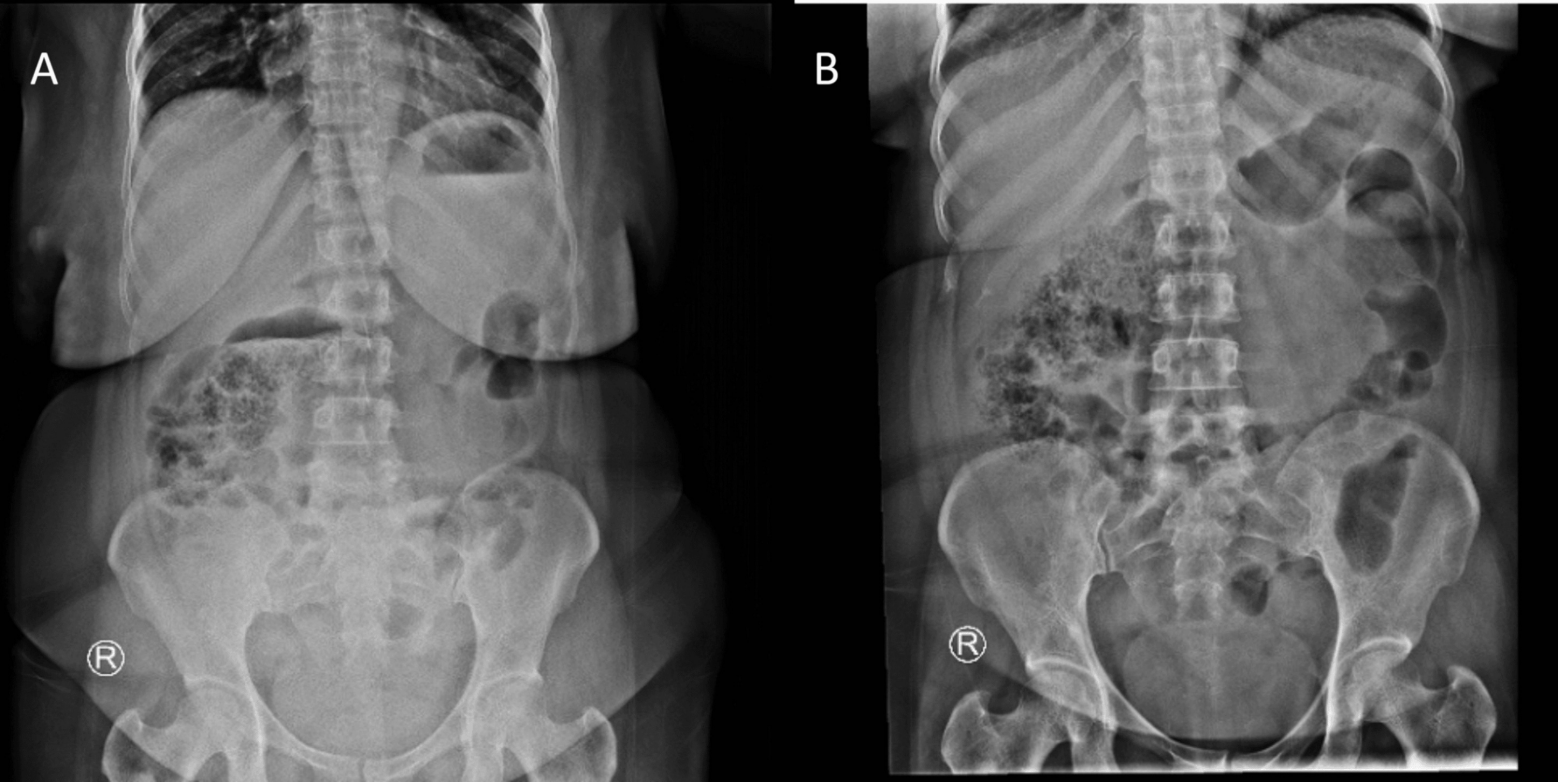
Second presentation abdominal X-ray (A) Erect; (B) Supine
